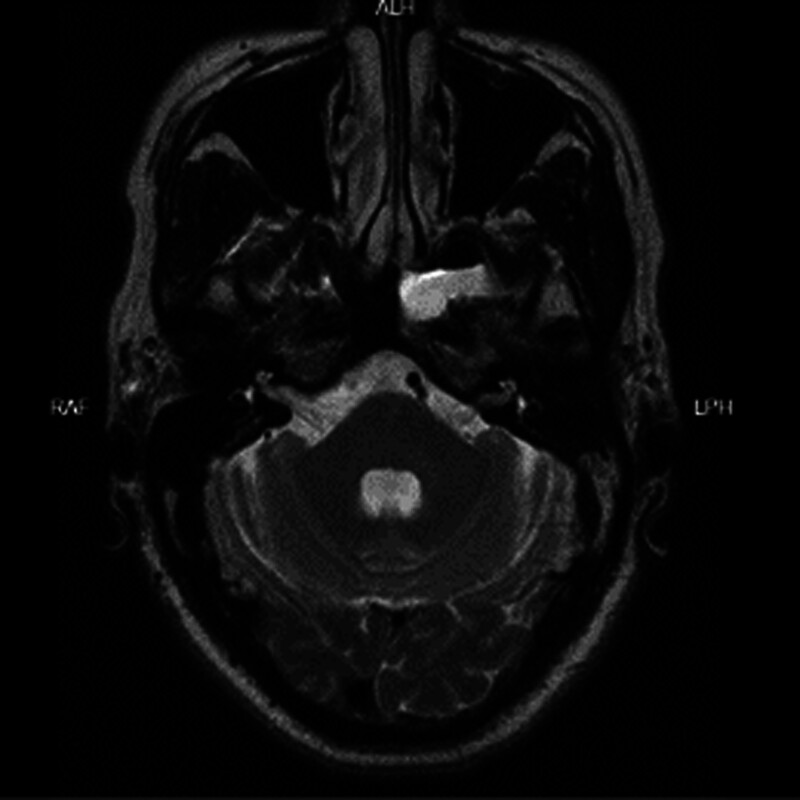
A 49-year old male patient with preoperative sinus MRI horizontal bitmap. MRI = magnetic resonance imaging.
